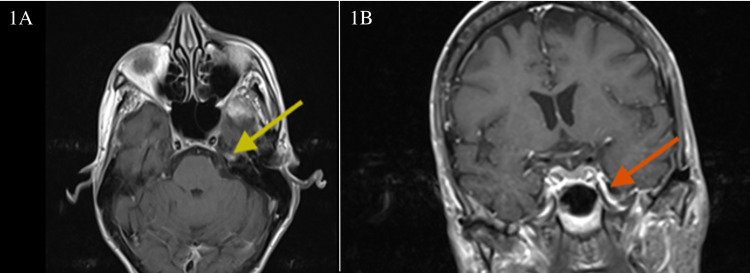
T1 post-contrast weighted MRI images of the brain 1A: Axial T1 post-contrast weighted MRI images with gadolinium enhancement of the semilunate Gasserian ganglion (yellow arrow). 1B: Coronal T1 post-contrast weighted MRI images demonstrating mandibular nerve enhancement in the foramen ovale (red arrow) MRI: magnetic resonance imaging
